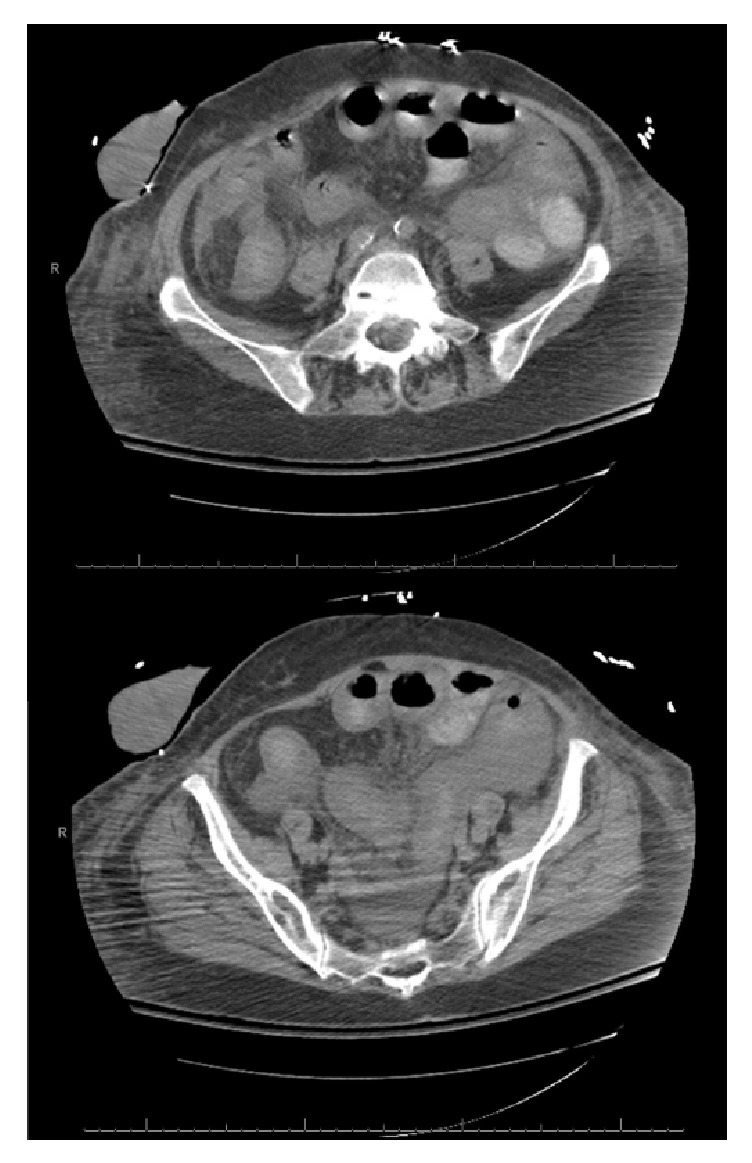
Second CT scan abdomen and pelvis two days later. Nonenhanced axial images showing diffuse small bowel dilation and intraperitoneal free fluid.
